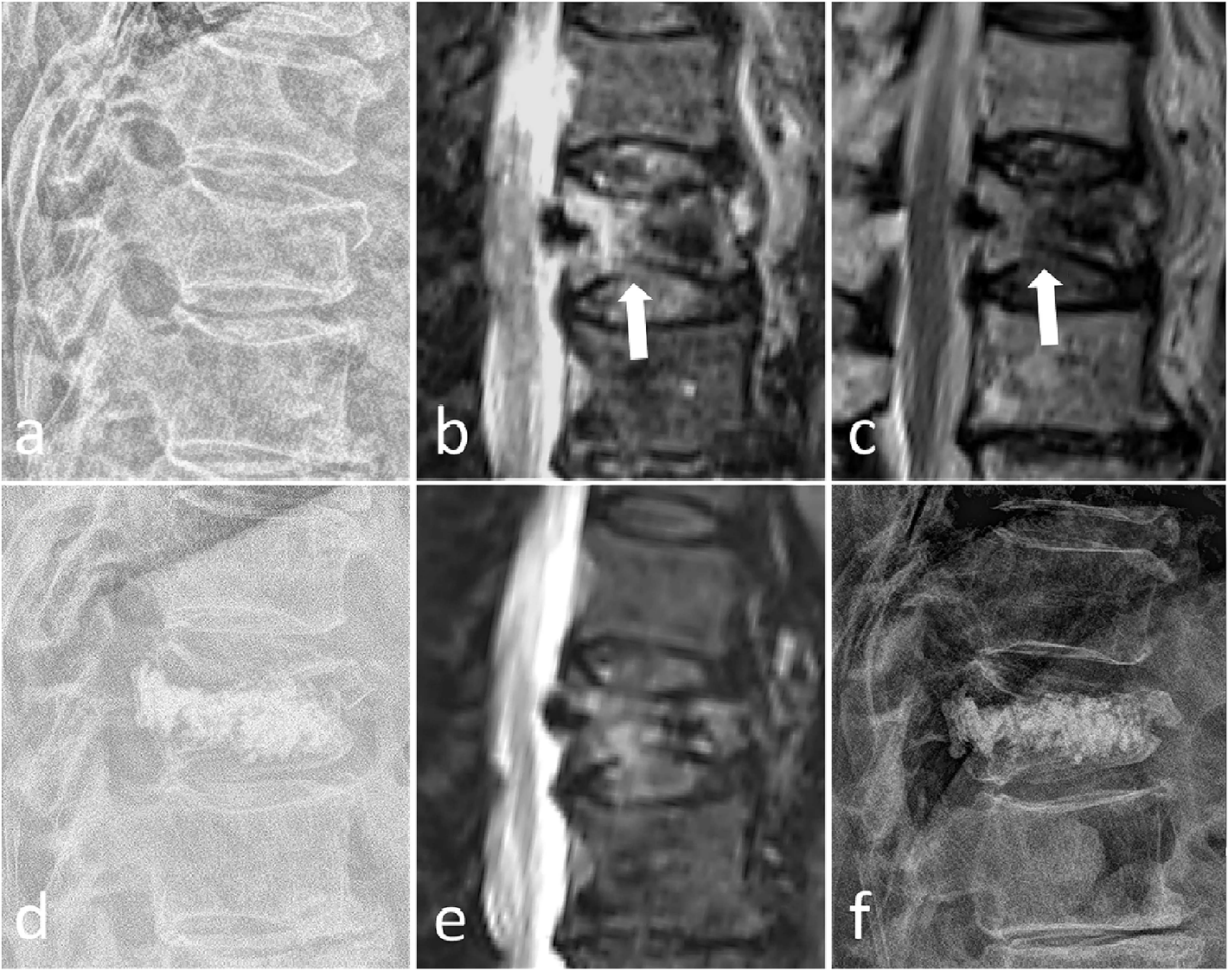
A, 85-year-old woman with refracture after PVP. Preoperative lateral radiographs, SPAIR, and T2W images (a-c) showed a T12 compression fracture with endplate cortical disruption (black arrow). Postoperative lateral radiographs (d) after 1 day showed NPEC on the lower and upper endplates. The SPAIR image and lateral radiograph 6 months after PVP (e, f) showed refracture of the cemented T12 vertebra with loss of height and bone marrow edema
